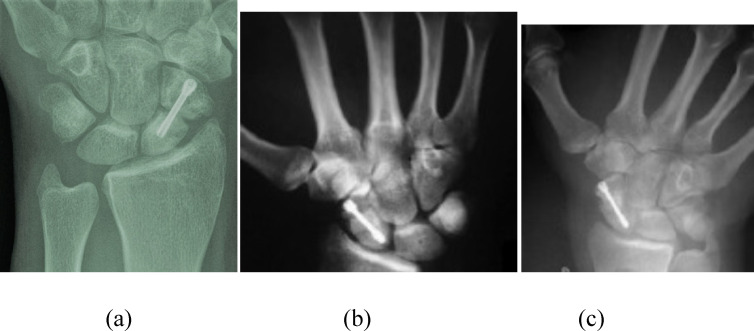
a) Preoperative PA wrist X-ray of non-united scaphoid fracture after fixation with Herbert screw. b) Postoperative X-ray after revision fixation using vascularized MFC graft and refixation. c) Follow-up X-ray showing full scaphoid union.
